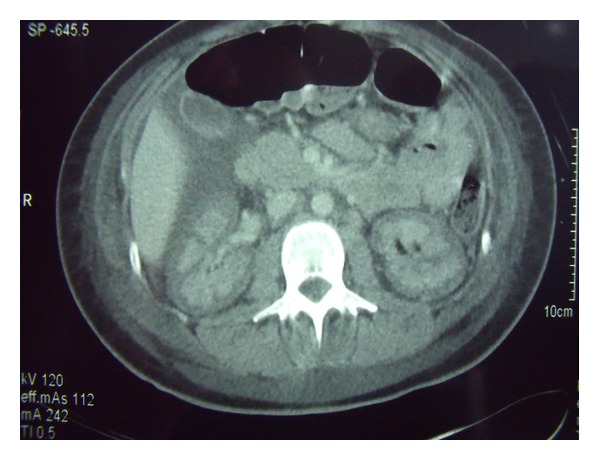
Computed tomography (CT) scan showing bilateral renal infarcts.
